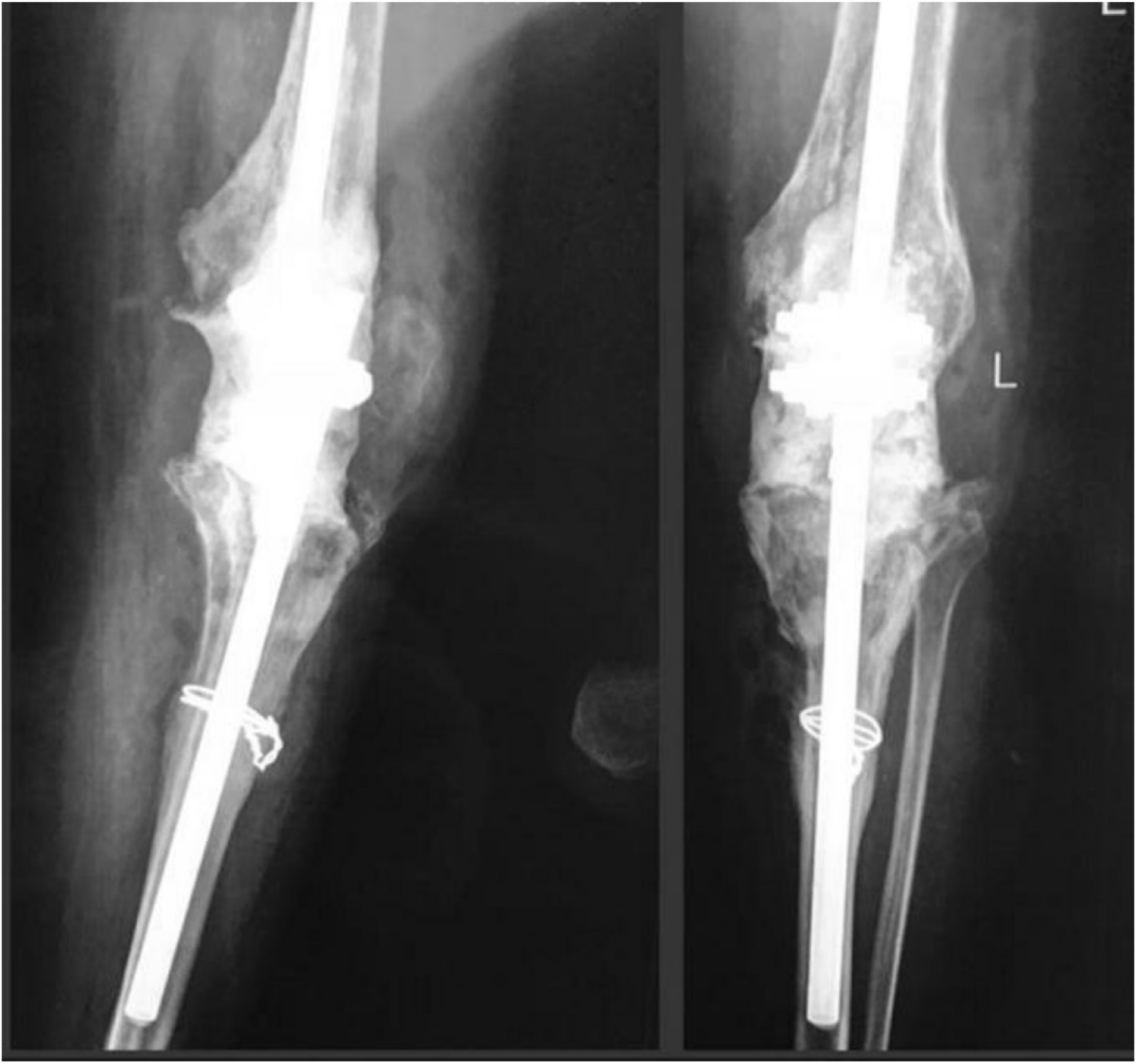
Anterioposterior and lateral postoperative knee radiograph. Patient with rheumatoid arthritis and osteoporosis.
